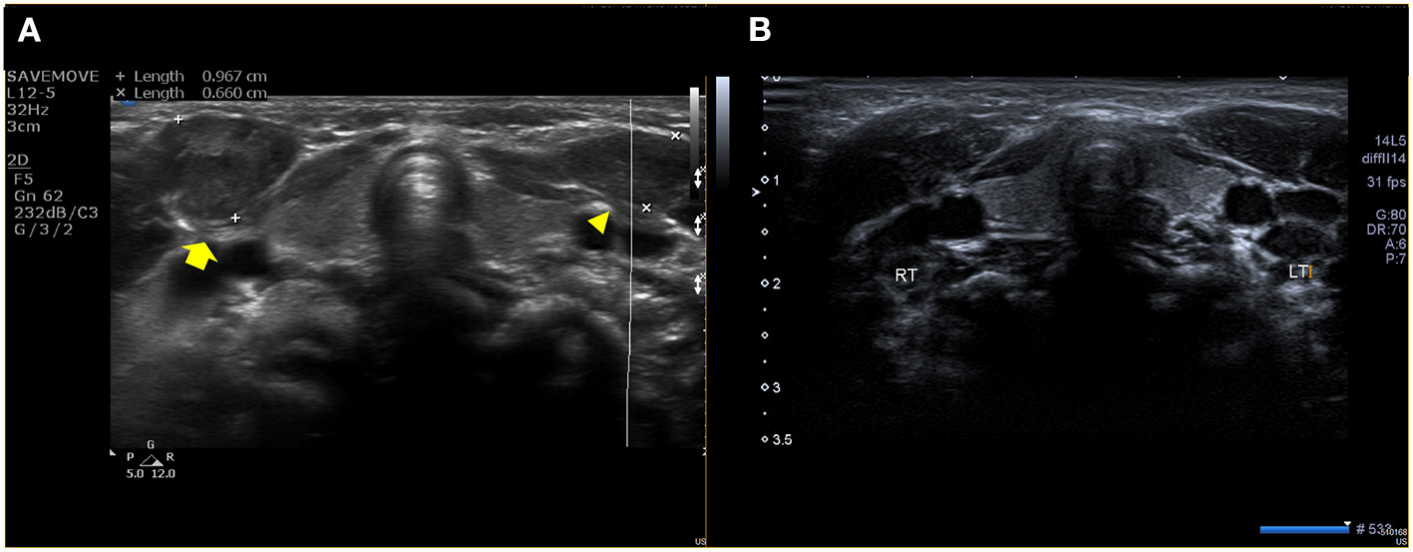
Ultrasonography of the right (yellow arrow) and left sternocleidomastoid muscles (arrowhead), revealing a fibromatosis colli in the right sternocleidomastoid, suggesting right CMT at the age of 3 months (A). A follow-up test at the age of 7 months showed the decreased size and improved echogenicity in the right sternocleidomastoid (B).
